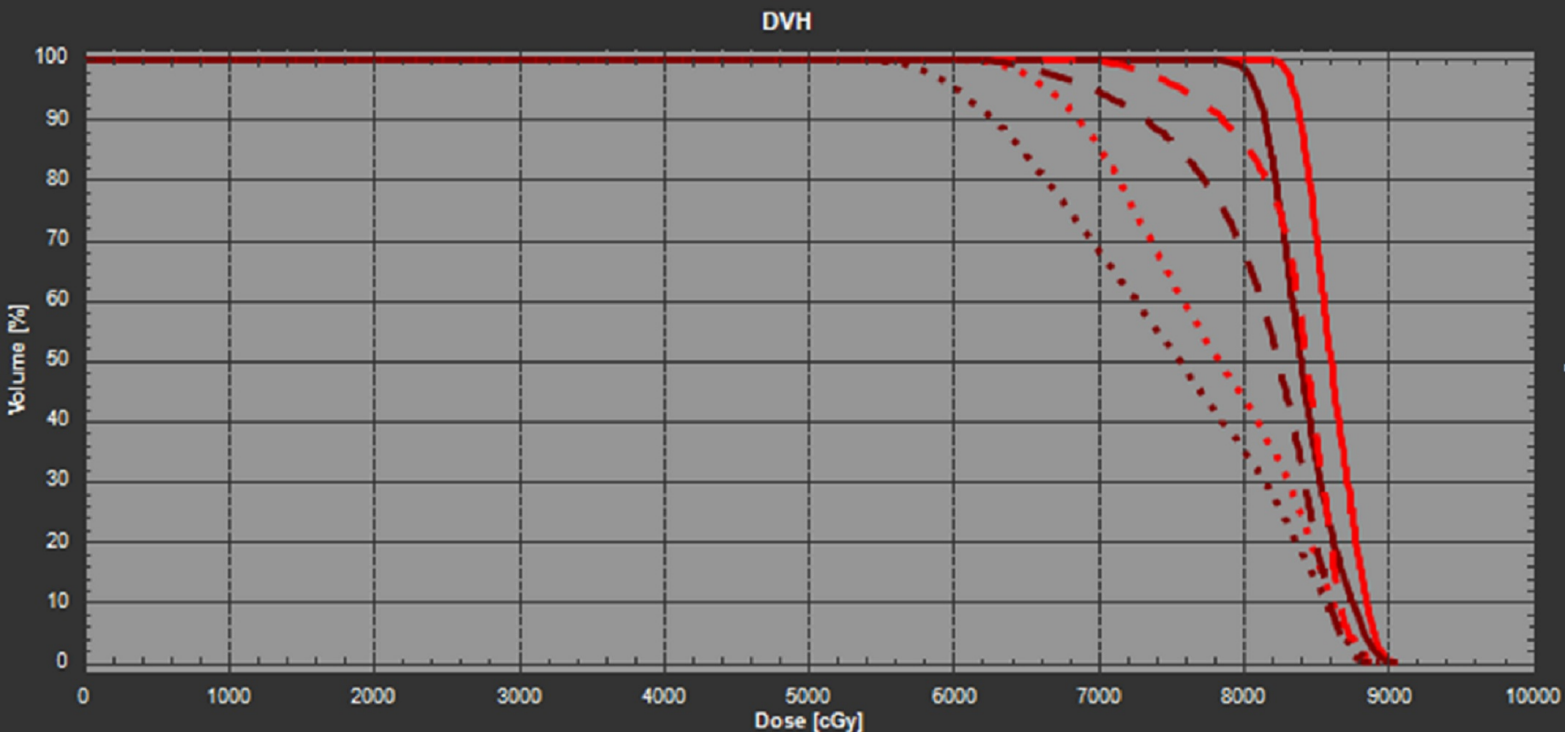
The DVH of the nominal plan in the planning CT (solid line), VFCT1 (dotted line), and VFCT2 (dashed line). The red lines denote the iGTV and the dark brown lines denote the CTV. CT: Computed tomography; CTV: Clinical target volume; DVH: Dose volume histogram; iGTV: internal gross target volume; VFCT: Verification CT.
